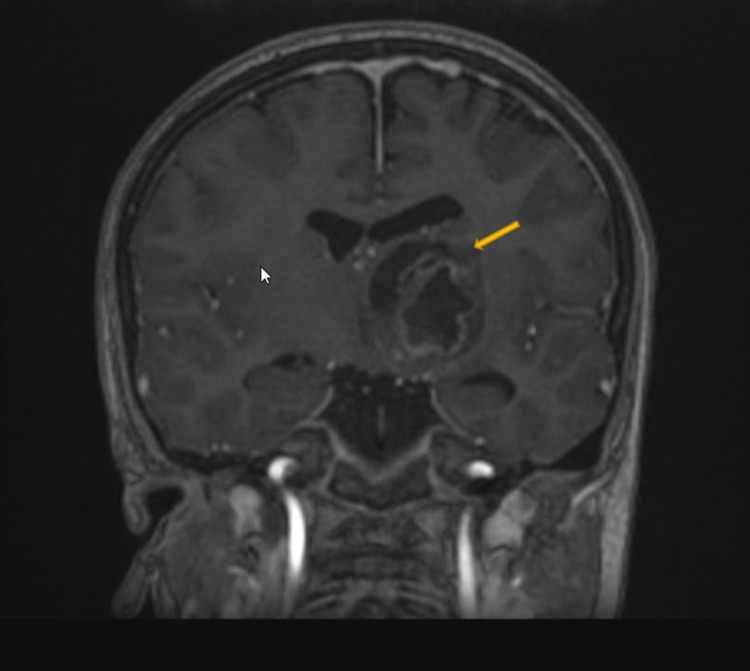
Preoperative coronal T1-weighted MRI and DTI with contrast The image shows left thalamic mass with heterogenous enhancement and mass effect on the third ventricle. DTI: diffusion tensor imaging
